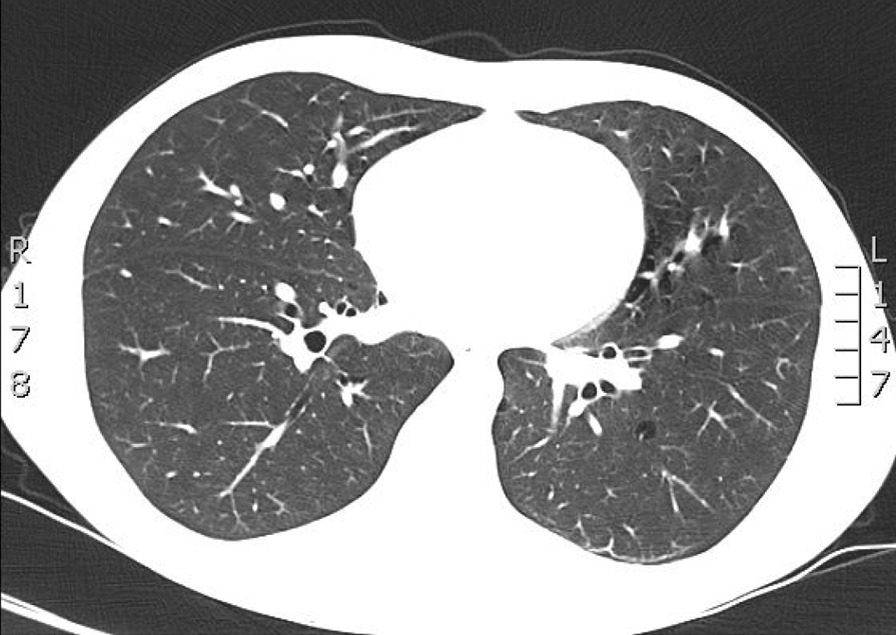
CT: multiple areas with ground-glass opacity and centrilobular nodules with a linear branching pattern (tree-in-bud sign)
